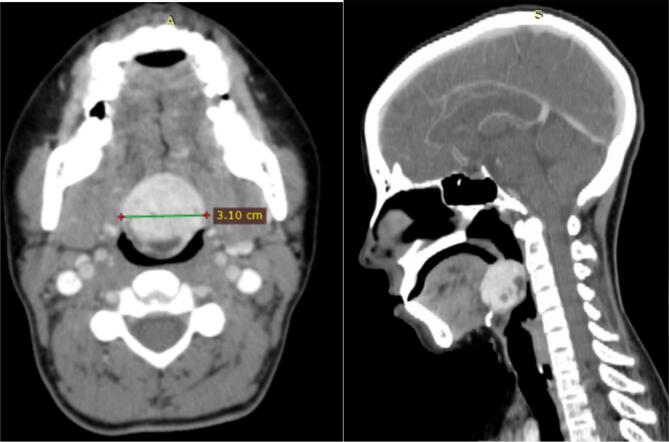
Post contrast standard Neck CT, there is a 3.10*3 cm mass hyperdense mass lesion at the level of the tongue base.
